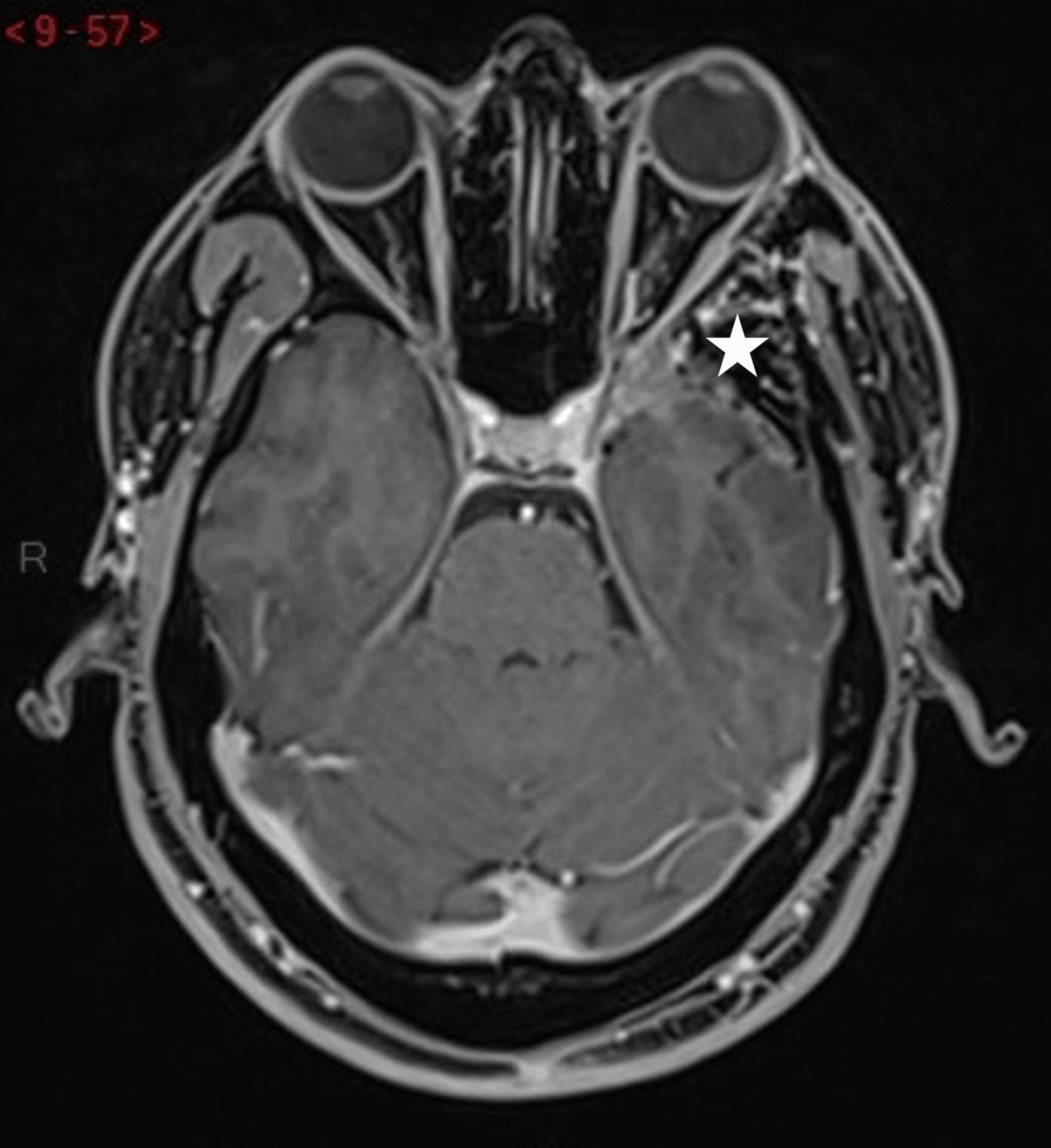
Postoperative axial T1-weighted brain MRI with gadolinium showing complete resection of the different components of the lesion (white star). Complete resection of the different components of the lesion (white star).
